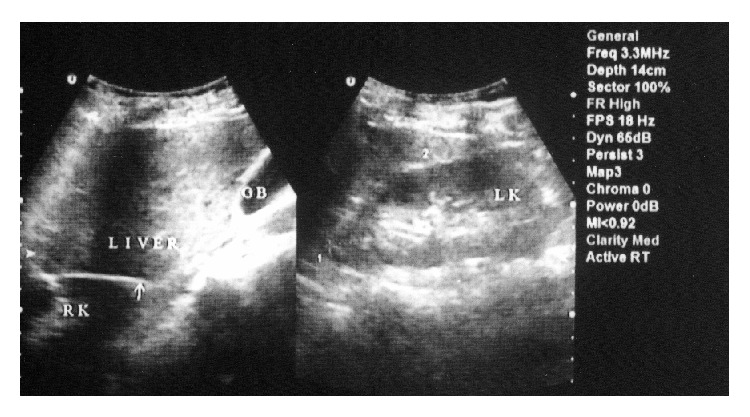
32-year-old man, diagnosed for ectopic kidney; ultrasonographic evaluation revealed an ectopic right kidney above the right hemidiaphragm. Both kidneys have normal size, echo pattern, and cortical thickness.
